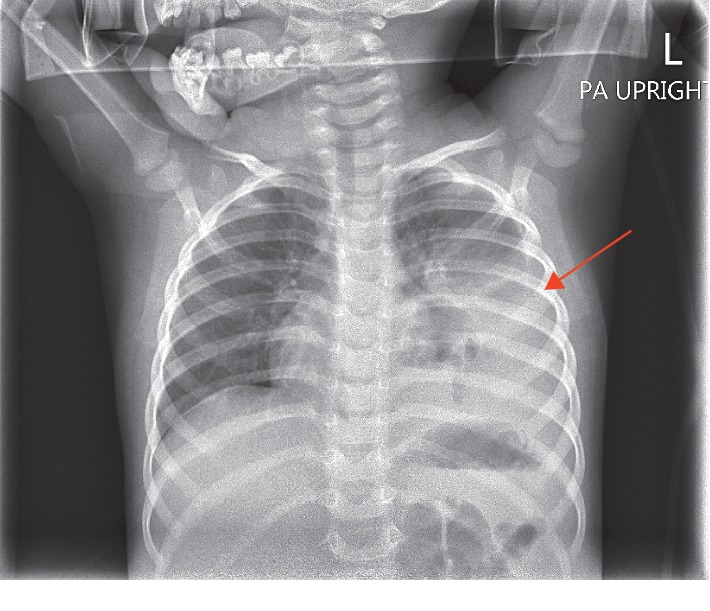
Posterioranterior (PA) chest X-ray demonstrating large consolidation in left lower lobe secondary to pneumonia vs. atelectasis with moderate-sized left pleural effusion noted.
